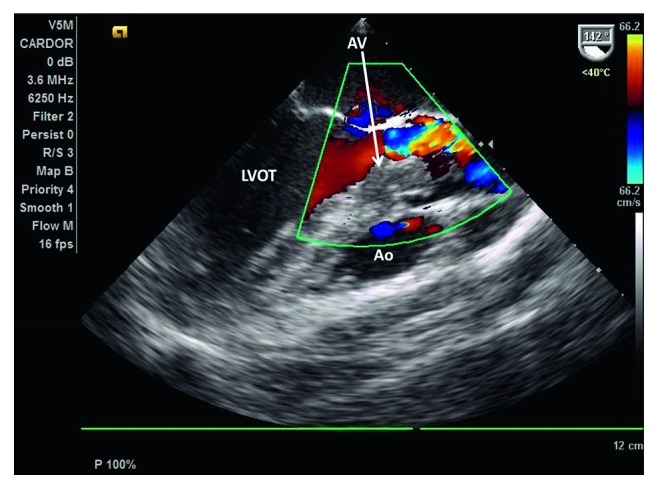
Doppler showing functional aortic stenosis and regurgitation.
